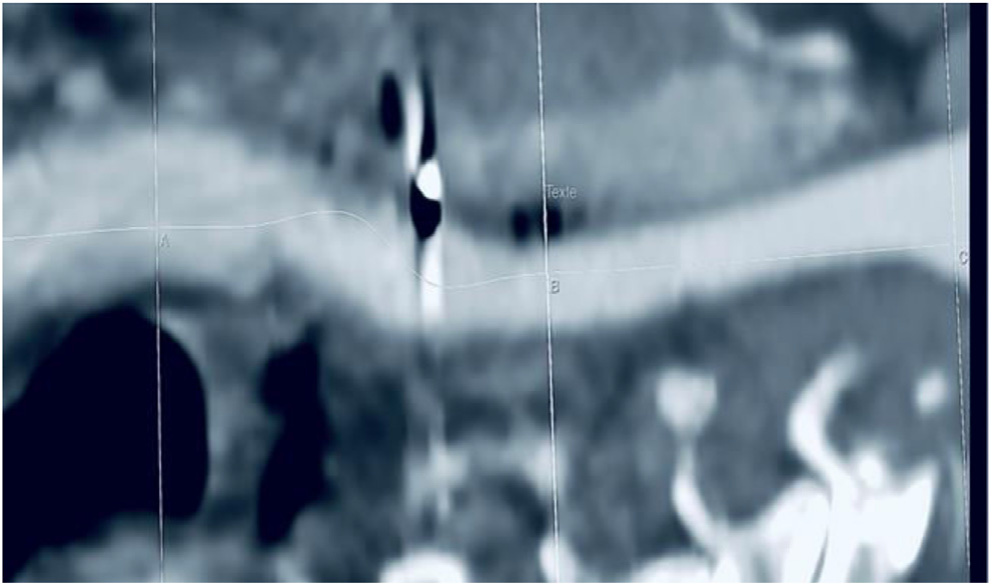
CTA centerline reconstruction of the right common carotid artery demonstrating a metallic pellet trajectory in close contact with the vessel wall, without evidence of luminal narrowing or contrast extravasation.
